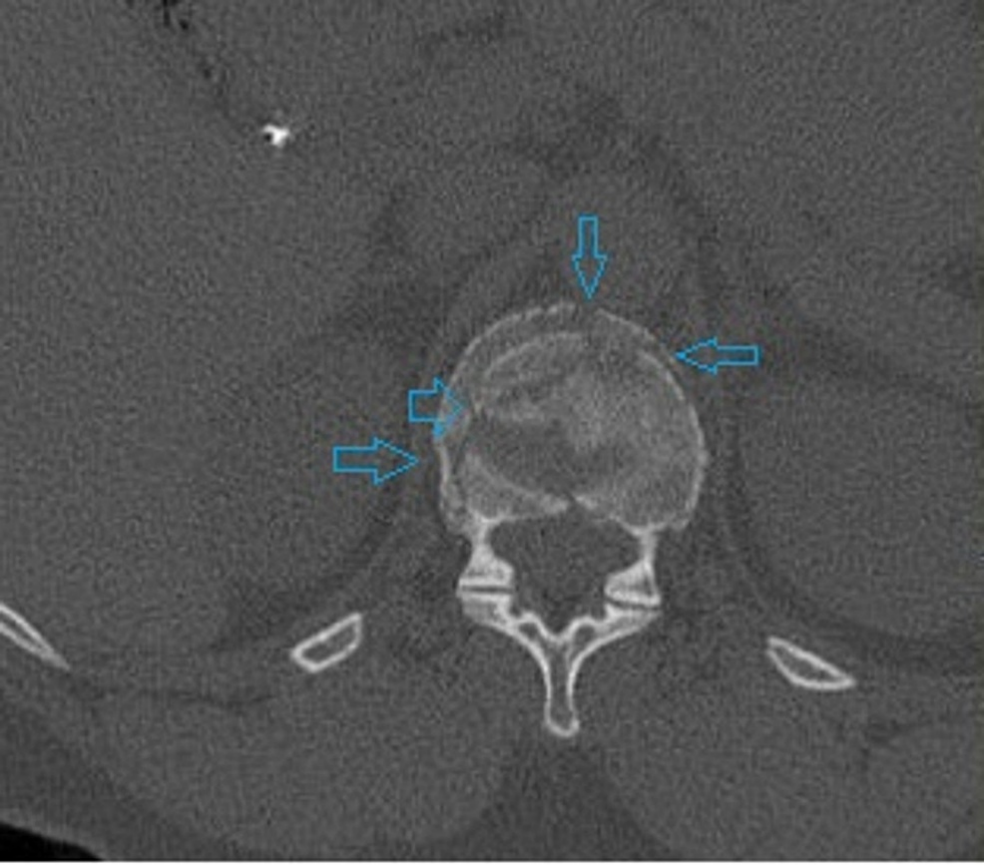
Axial computed tomography image. Small blue arrows demonstrate the comminution of the fractured vertebral body.
